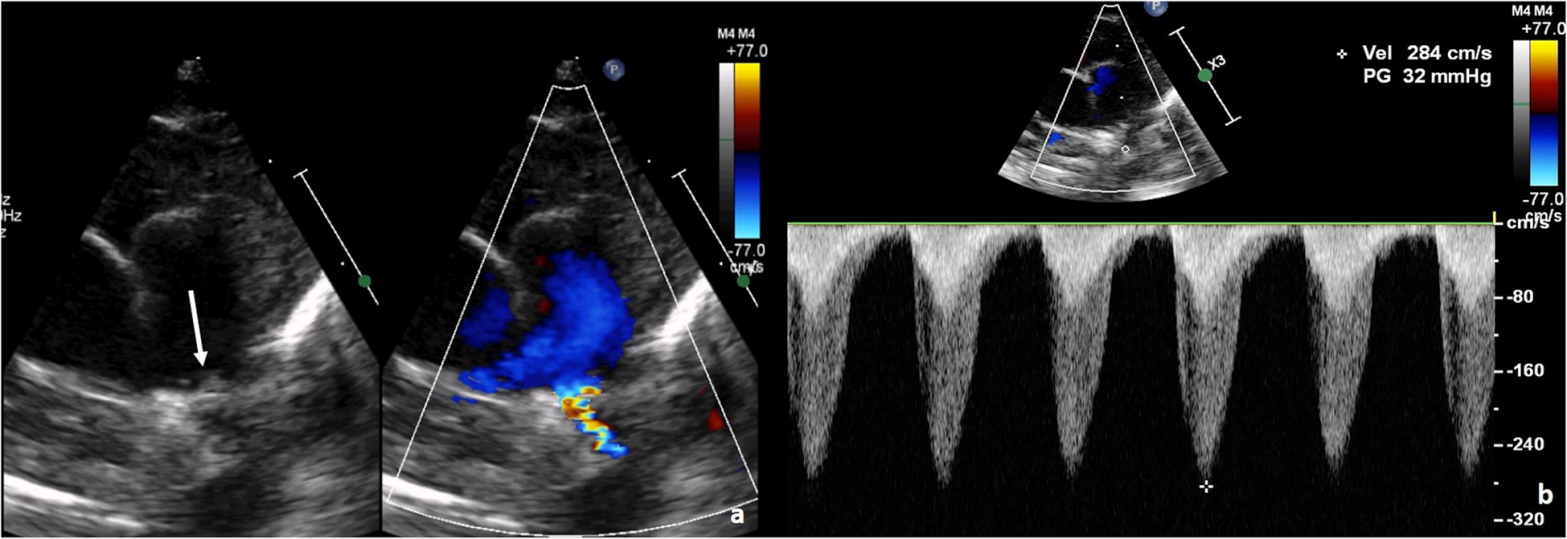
a Echocardiogram with colour Doppler in parasternal short axis view showing turbulent flow across LPA along with luminal narrowing (arrow). b Echocardiogram with continuous wave Doppler showing gradient across LPA
